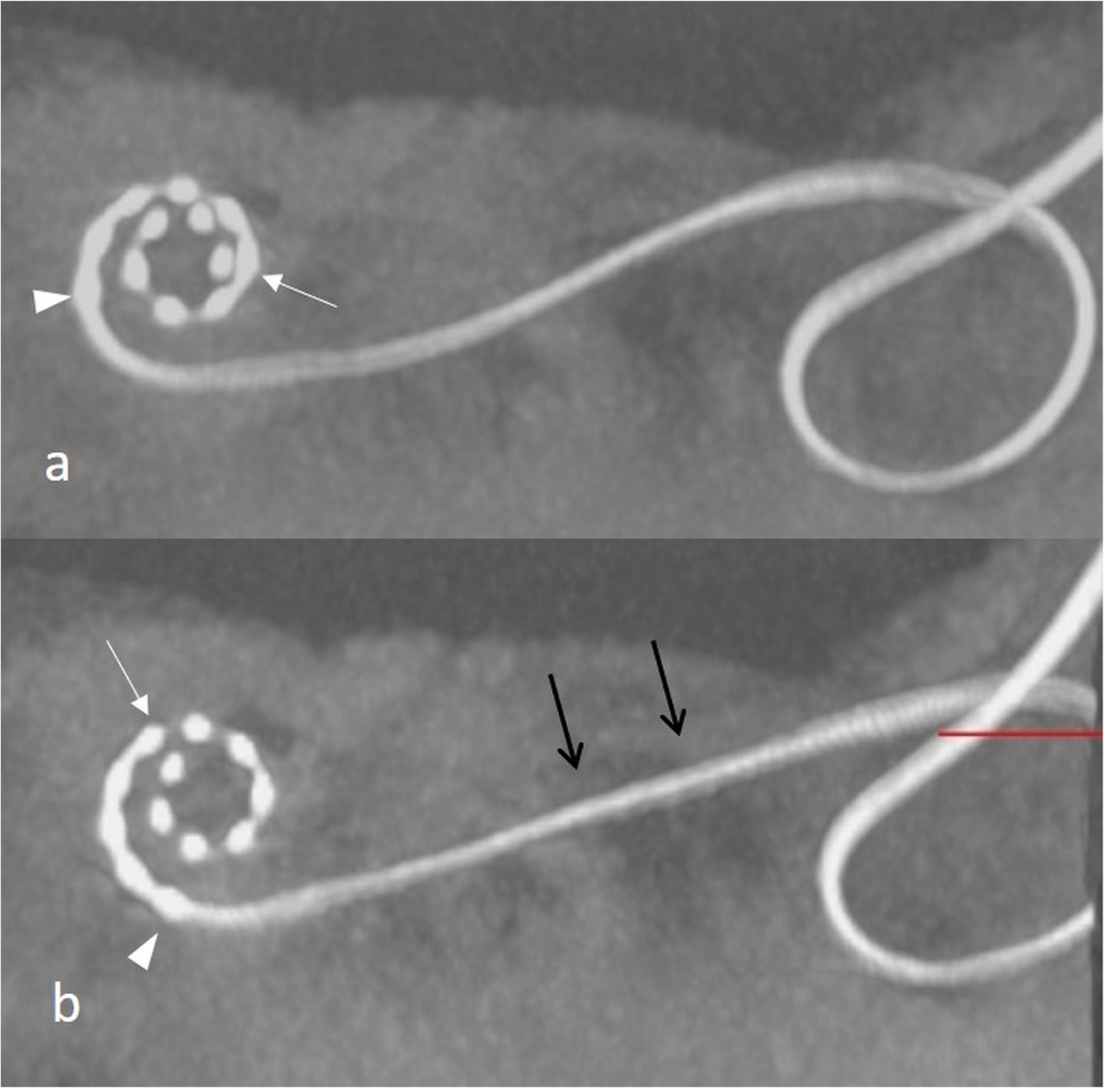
A 53-year-old male patient, with a complete malfunction of the CI and pain 3 months after CI surgery. CBCT paraaxial maximum intensity projection image shows initial overinsertion of the electrode array (a). The control scan shows back extrusion of the electrode array with migrated positions of the electrode tip (arrow) and basal electrode element (arrowhead) and straightened electrode array (black arrows) in the mastoidectomy cave (b)
